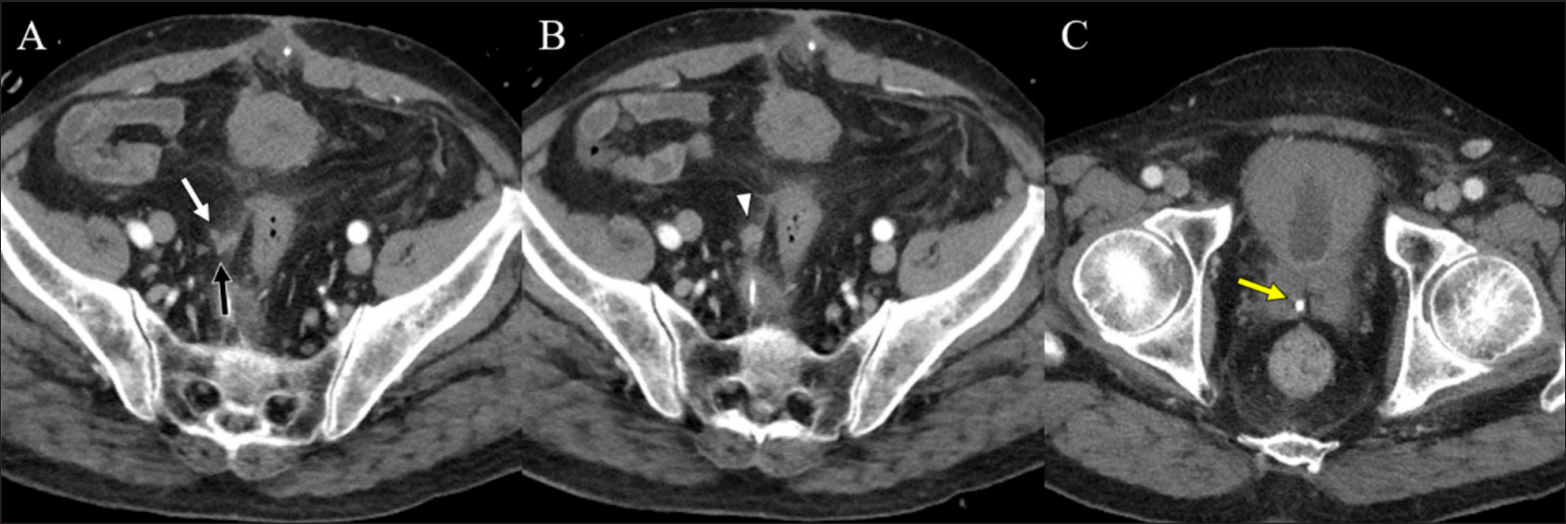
Follow-up CT images were taken after 11days. Axial images (A, B) show no abscess other than enhancing soft tissue (arrowhead) around the defect site of the VD wall. An axial image (C) shows a remnant stone at the distal VD. (white arrow: proximal VD, black arrow: distal VD, yellow arrow: VD stone).
